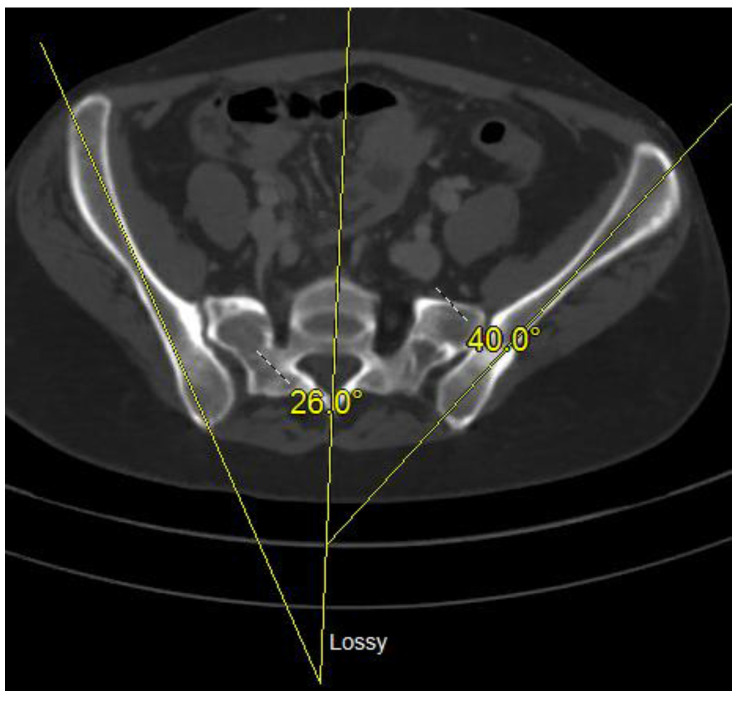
Axial CT image of a pelvic malunion with internal rotation deformity of the right hemipelvis.
